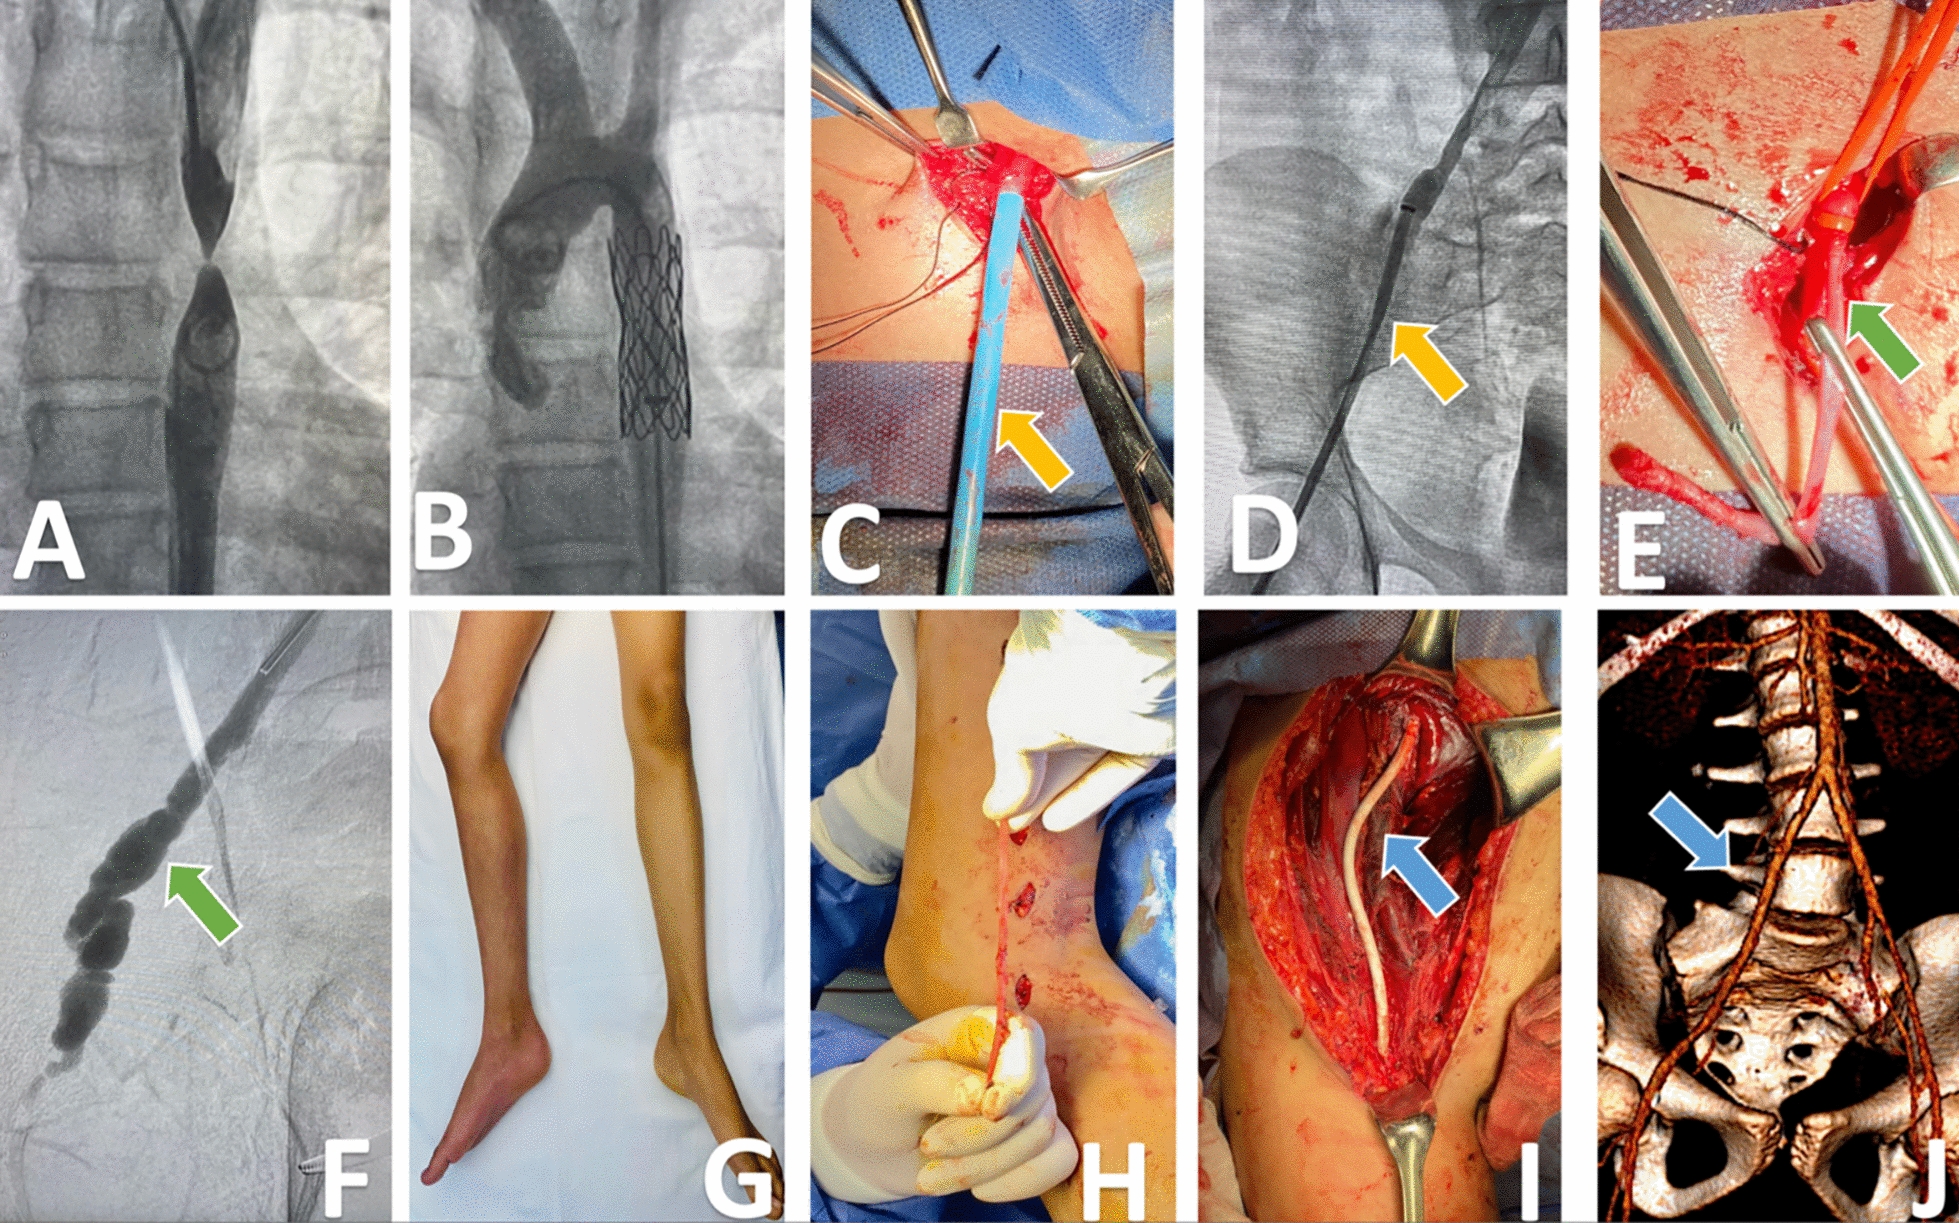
From left to right and chronologically, the images of the case of a 12-year-old female patient who underwent aortic thoracic stent placement due to aortic coarctation (a and b), with the impossibility to remove the 12 Fr sheath once the procedure was completed. c Yellow arrow signaling the sheath inside the common femoral artery, d the tip of the sheath in the common iliac artery, yellow arrow signaling a point of narrowing in the external iliac artery (EIA), e green arrow indicates eversion of the intima and media layers of the external iliac artery through the femoral puncture once the introducer was removed, f green arrow indicates an arteriography image of EIA pseudoaneurysm with imminent rupture risk after sheath removal. g Right lower limb with cyanosis. h Great saphenous vein harvested and unsuitable as a graft. i Blue arrow indicates the interposition of ePTFE 6 mm graft from the internal iliac artery to the common femoral artery as a method of vascular reconstruction, j 3D reconstruction of postoperative abdominopelvic Angio CT, where the patency of the PTFE graft is evident and signaled with a blue arrow
